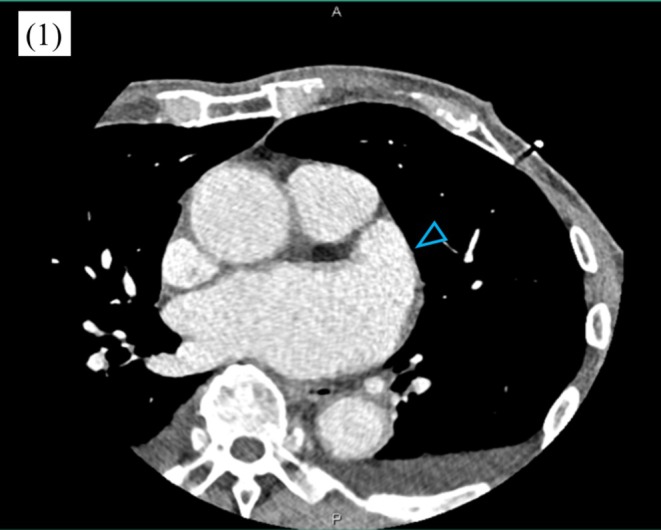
Contrast‐enhanced cardiac computed tomography prior to ablation showing no filling defects in the left atrium (LA) or left atrial appendage (LAA). The LAA is indicated by the blue arrow.
